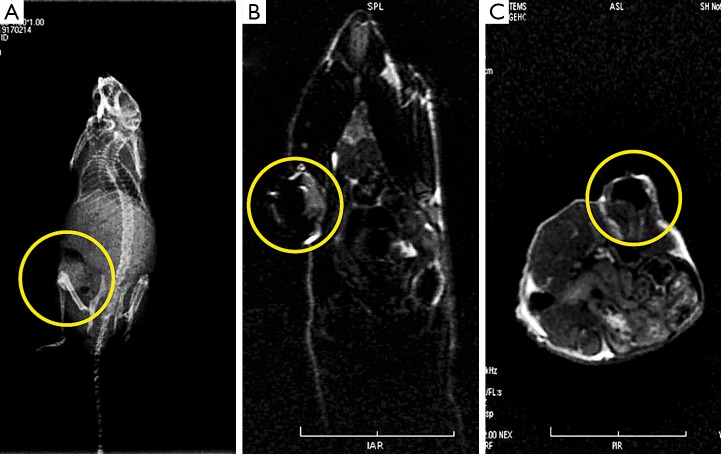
JenyX-ray and MR imaging of *in vivo* gastric cancer. (A) X-ray imaging. (B) MR T_1_ imaging. (C) MR T_2_ imaging.
